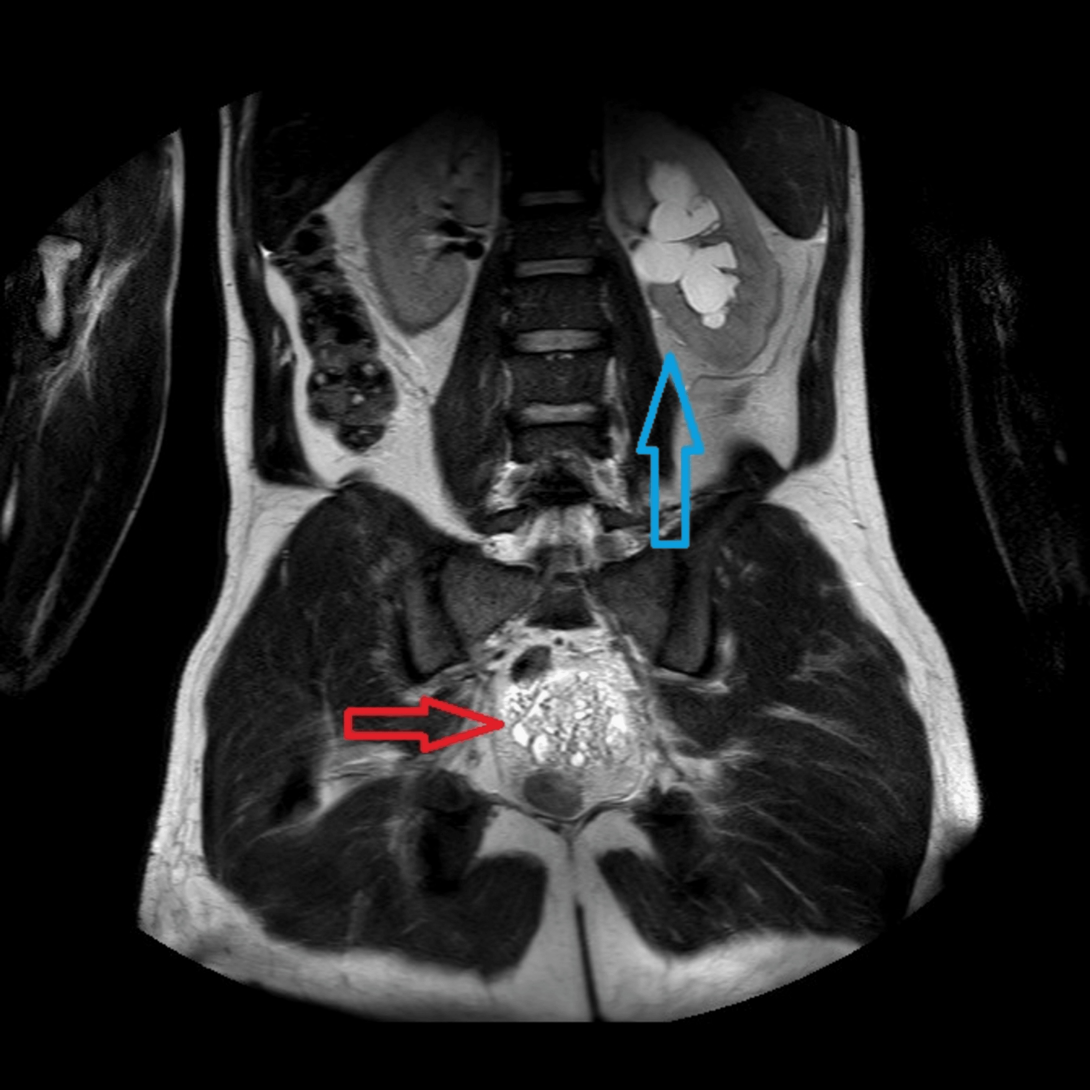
Abdominal MRI scan using T2-weighted turbo spin echo in the coronal plane showing neoplasm infiltrating the prostate (red arrow) and hydronephrosis of the left kidney (blue arrow).
